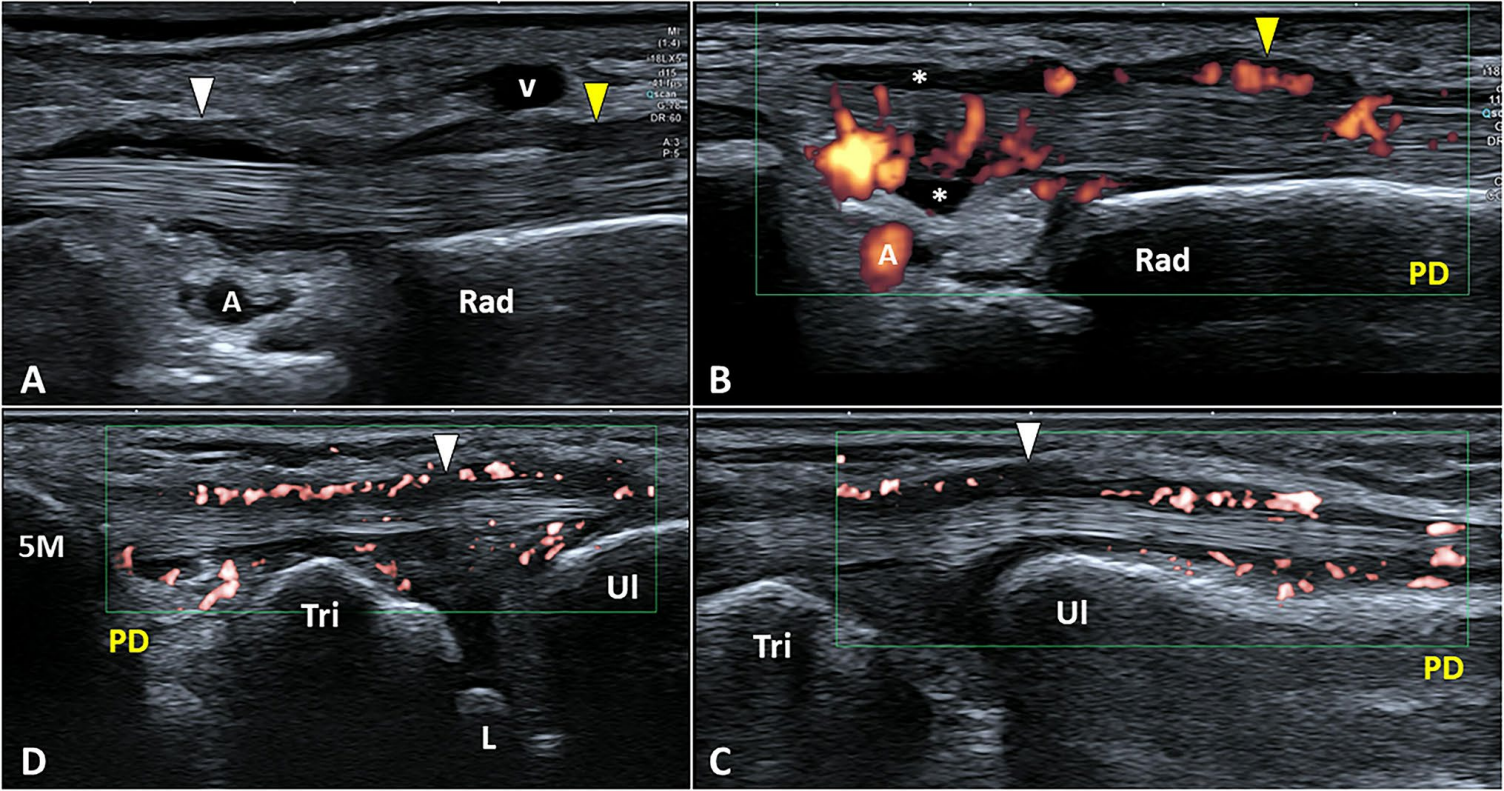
Thickening of the fibrous retinaculum *(yellow arrowhead)*, sheath effusion *(white asterisks)*, tenosynovial hypertrophy *(white arrowhead)* with hypervascularization of the first extensor compartment is visualized in a padel player with radial wrist pain (A, B). Likewise, tenosynovitis of the extensor carpi ulnaris tendon with multiple vascular signals within the hypertrophic synovial sheath *(white arrowhead)* can be observed on the ulnar side (C, D). *Rad* radius, *A* artery, *V* vein, *PD* power doppler, *Ul* ulna, *Tri* triquetrum, *L* lunate, *5M* fifth metacarpal bone
